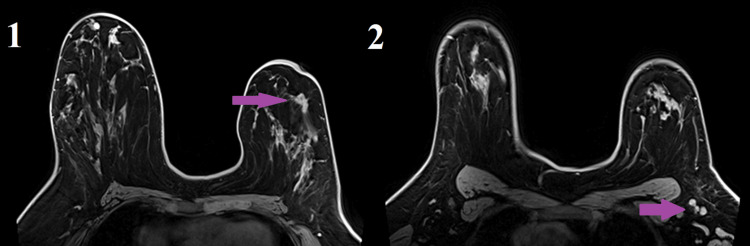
MRI in axial view: Pane 1: an irregular spiculated enhancing mass (purple arrow) at 2-3 o’clock 2 cm from the nipple; Pane 2: abnormal axillary lymph nodes with suspected cortical thickening suspicious for nodal metastasis (purple arrow).
